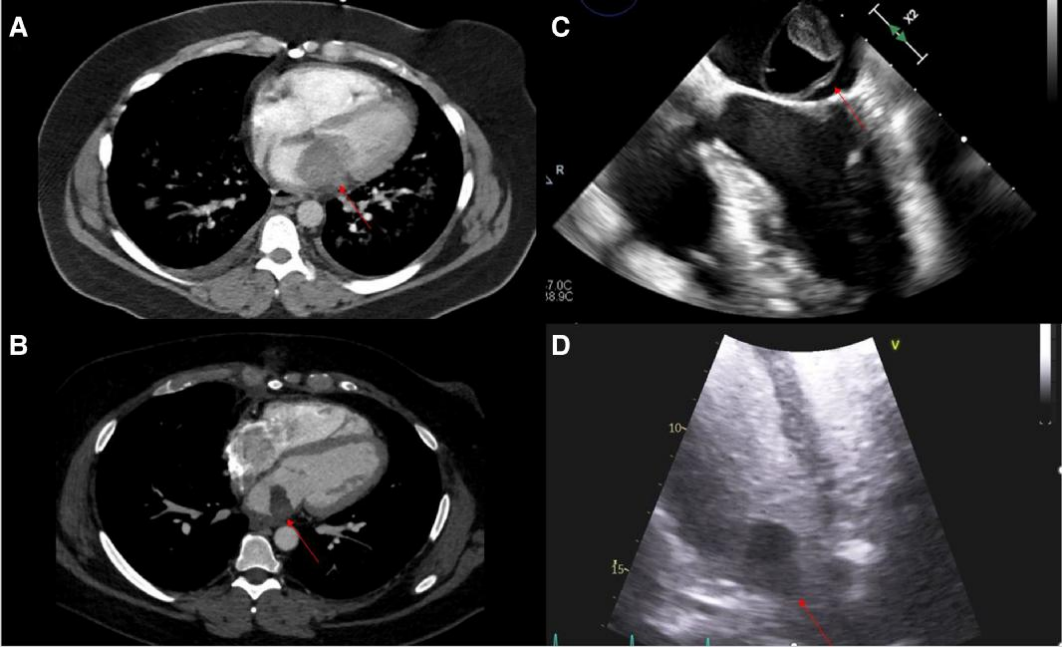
CT and echocardiographic images. (*A*) Initial CT pulmonary angiogram axial view demonstrating left atrial mass (arrow) and right pleural effusion. (*B*) Repeat CT pulmonary angiogram axial view 1 month later demonstrating recurrence of left atrial mass (arrow). (*C*) Intraoperative transoesophageal echocardiogram four-chamber view demonstrating left atrial mass. (*D*) Transthoracic echocardiogram apical four-chamber view demonstrating left atrial mass.
